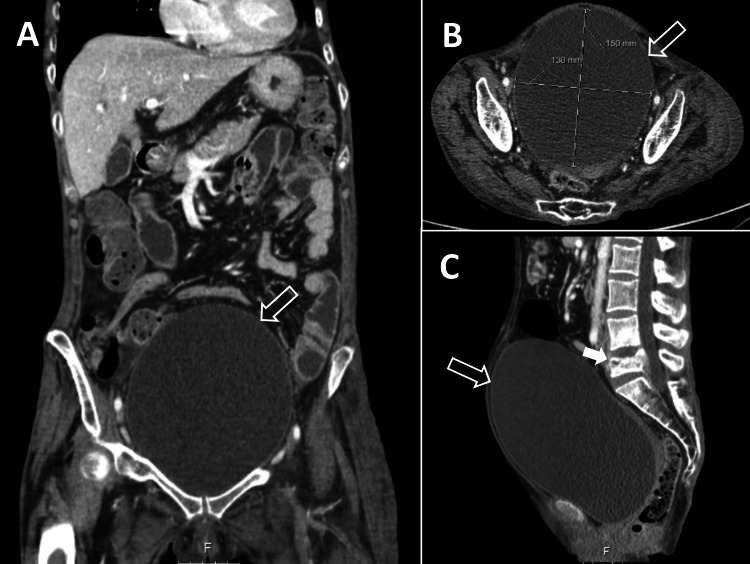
Abdominal CT scan. A: coronal view showing a hyperdistended bladder (void arrows); B: horizontal view with bladder measurements; C: sagittal view showing bladder hyperdistention and L5 compression fracture (white arrow).
